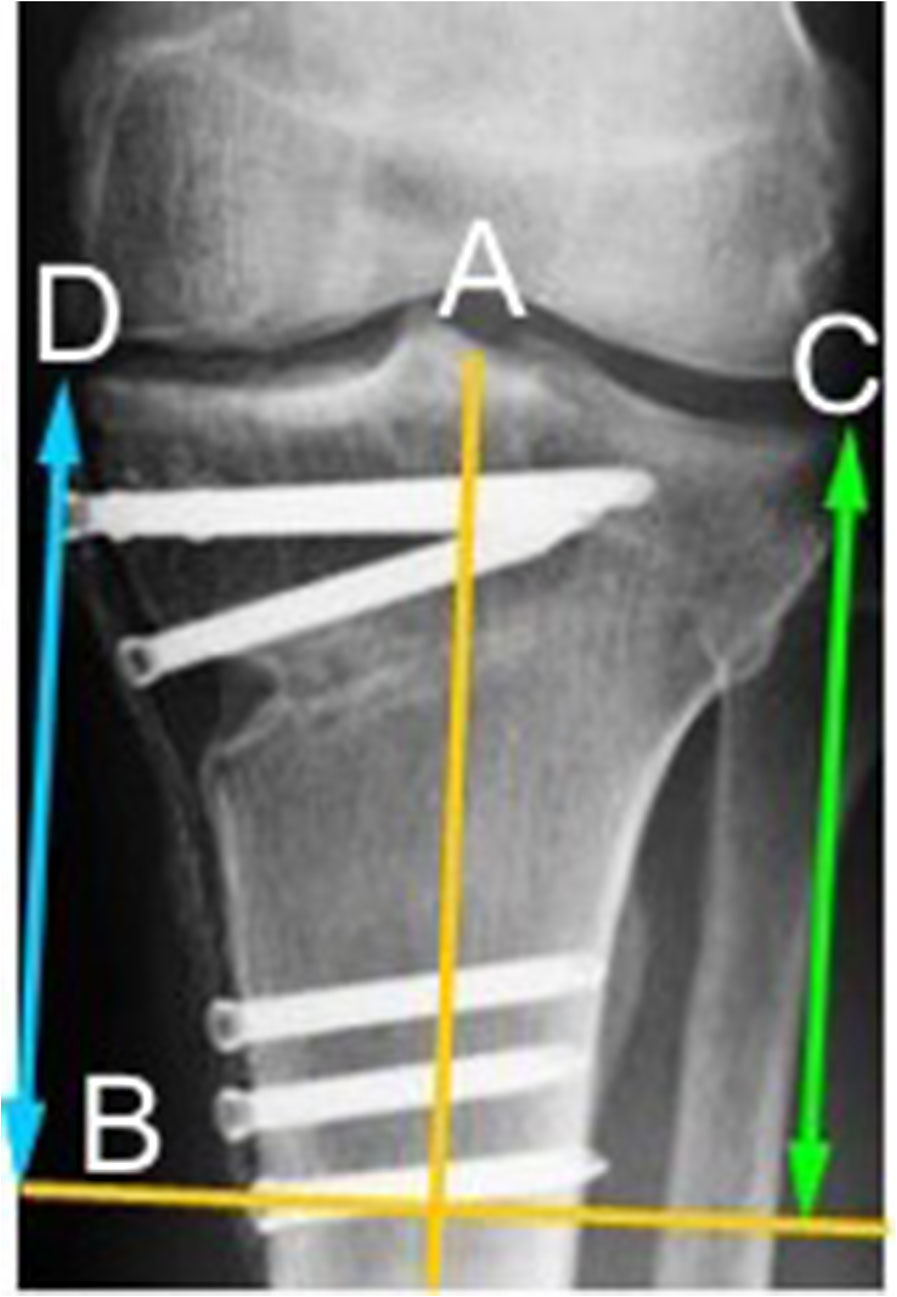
Measurement of loss of correction after owHTO on posterior-anterior radiographs. Line *A*: anatomical axis of the tibia; Line *B*: perpendicular to A through the center of the head of the lowest screw; *C*: lateral joint line edge of the tibia; *D*: medial joint line edge of the tibia. Loss of correction was determined by comparing the distance from point *C*, respectively point *D*, to line *B* in the radiographs after 4 weeks and 12 months
